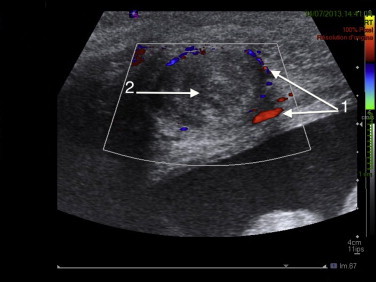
Doppler USG of the anterior abdominal area. (1) Circumferential vascularity. (2) Absence of flow.
